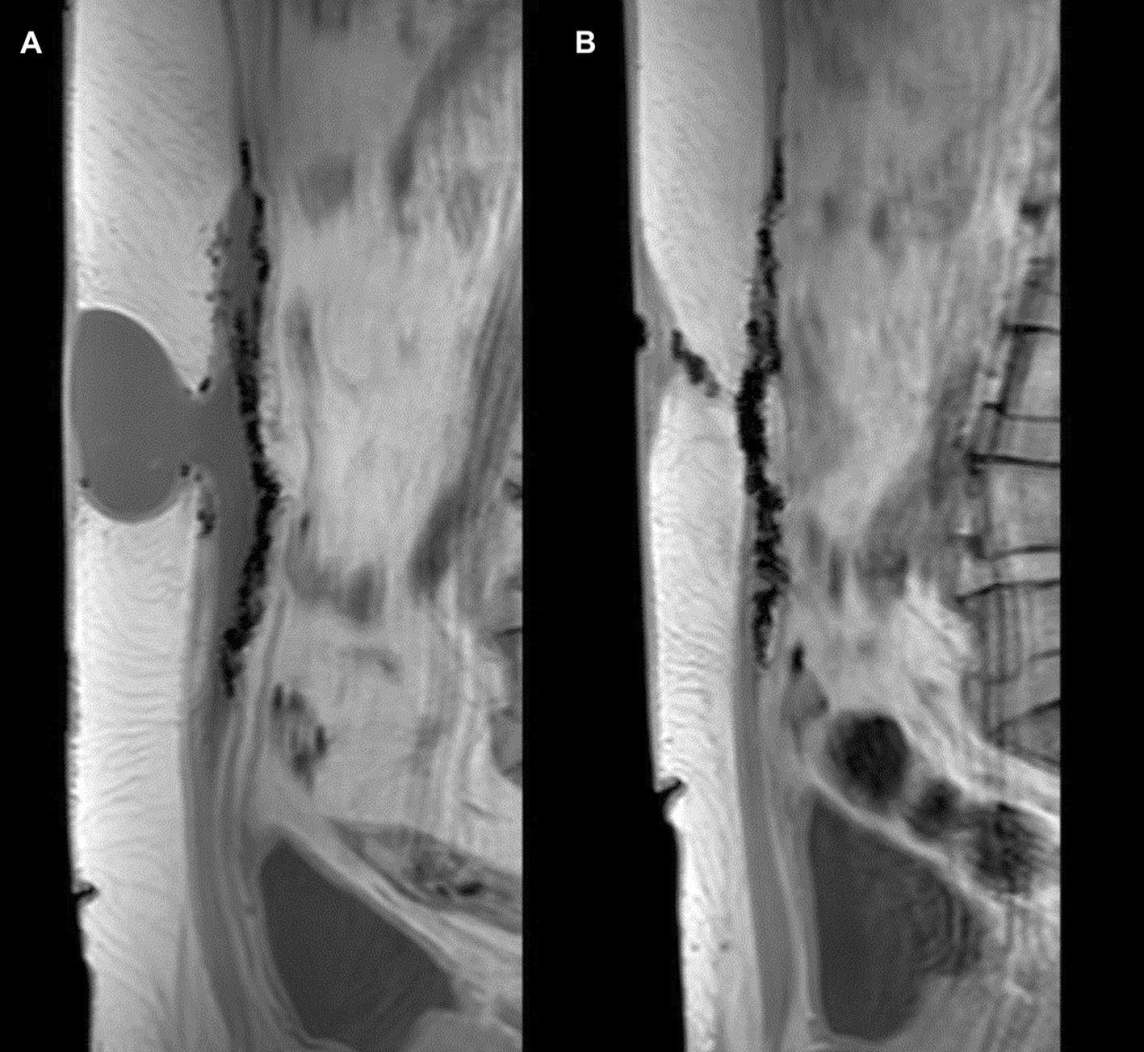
3DT1 MRI imaging in a patient who had a robot-assisted minimal invasive retrorectus ventral hernia repair using an iron-oxide-loaded PVDF mesh A MRI image at 1 month showing a postoperative seroma and a dehiscence of the hernia defect closure after an episode of heavy coughing B No residual seroma on MRI at 13 months postoperatively
